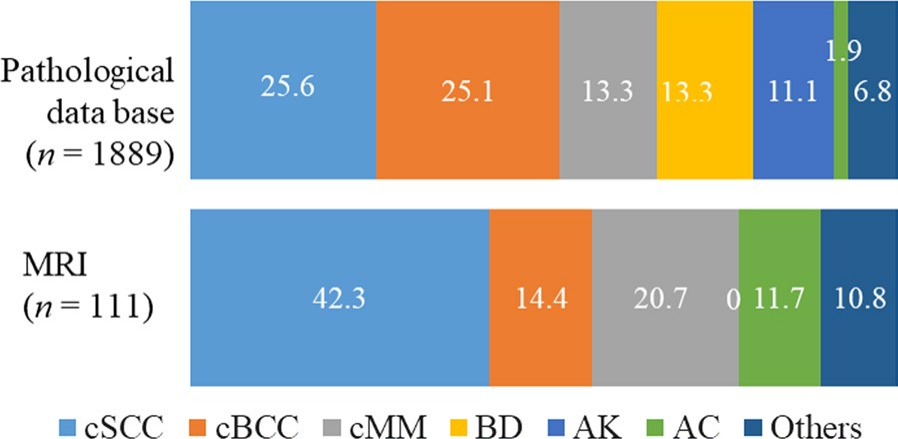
Pathological and MRI database in our institution. SCC = squamous cell carcinoma, BCC = basal cell carcinoma, MM = malignant melanoma, BD = Bowen’s disease, AK = actinic keratosis, AC = appendageal cancer
